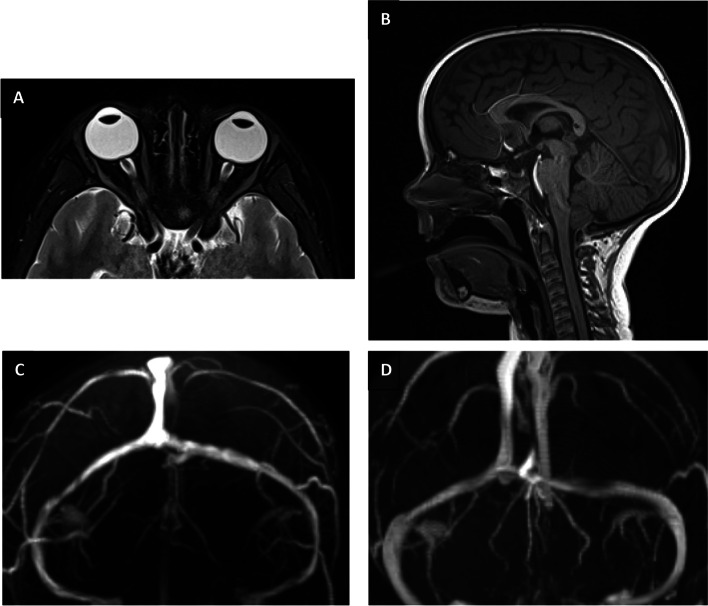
Magnetic resonance brain imaging demonstrated features consistent with intracranial hypertension. A T2-weighted with fat suppression axial image demonstrating flattening of the sclera of both globes posteriorly with papilloedema and distension of the optic nerve sheaths. B T1-weighted sagittal image demonstrating intrasellar arachnoid herniation. The pituitary gland and stalk were normal in appearance. C Time of flight MR venogram demonstrating bilateral stenosis of the lateral segments of the transverse sinuses, which resolves after treatment (D)
